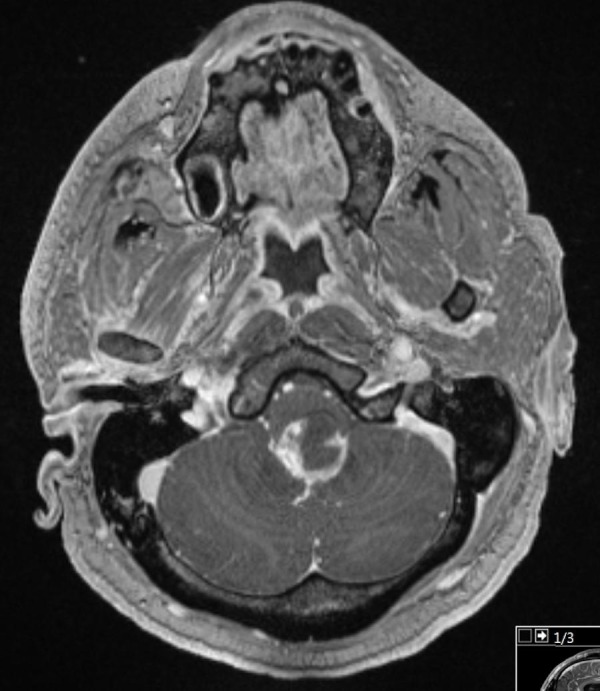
Preoperative magnetic resonance imaging (MRI). Post-gadolinium T1 MRI of the brain showing the enhancing lesion in the midline.
